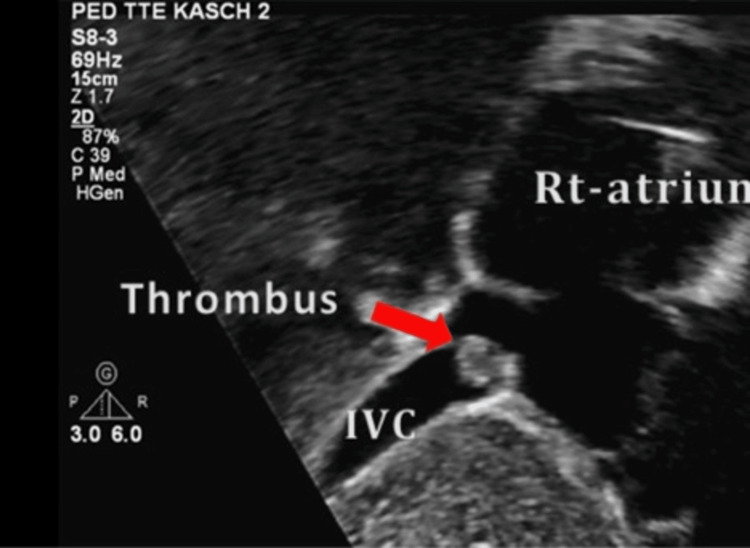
Echocardiography showing a thrombus at the junction of the inferior vena cava and right atrium
